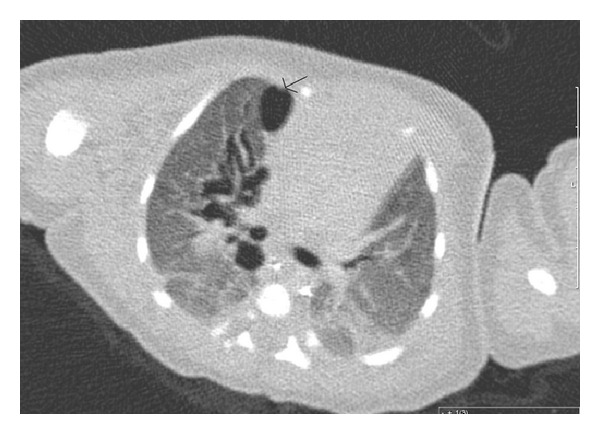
Representative slice from CT scan shows loculated pneumomediastinum (arrow) and multiple branching lucencies in a perivascular distribution, compatible with PIE.
